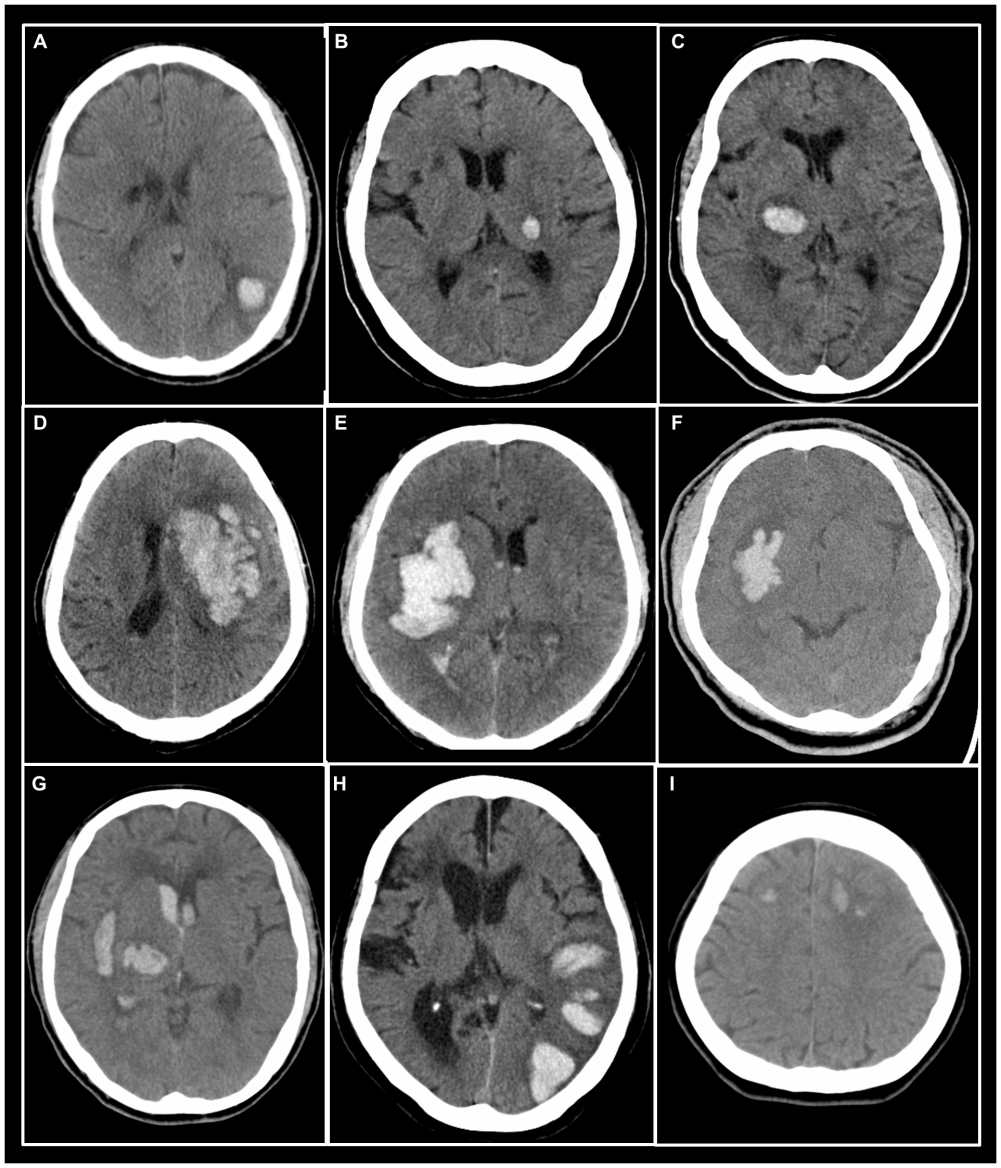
Axial brain CT images demonstrating regular hematoma shapes (A–C) and irregular hematoma shapes (D–I). Regular hematoma shape implies roundish (A) or ovoid (B & C) shape with smooth margin, while irregular hematoma shape refers to pleomorphic contour (D–F), separated adjacent hematomas (G & H) and multicentric hematomas (I).
